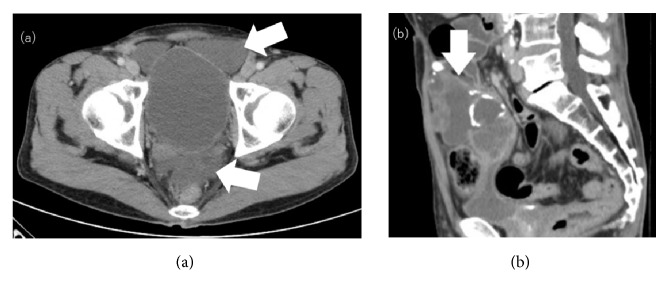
(a) Abdominal computed tomographic image taken after five cycles of gemcitabine and cisplatin (GC). Abdominal fluid was detected (white arrows). (b) Abdominal computed tomographic image taken after FOLFIRI (*ℓ*-leucovorin + 5-fluorouracil + irinotecan). The tumor size had decreased to 7 cm (white arrow).
